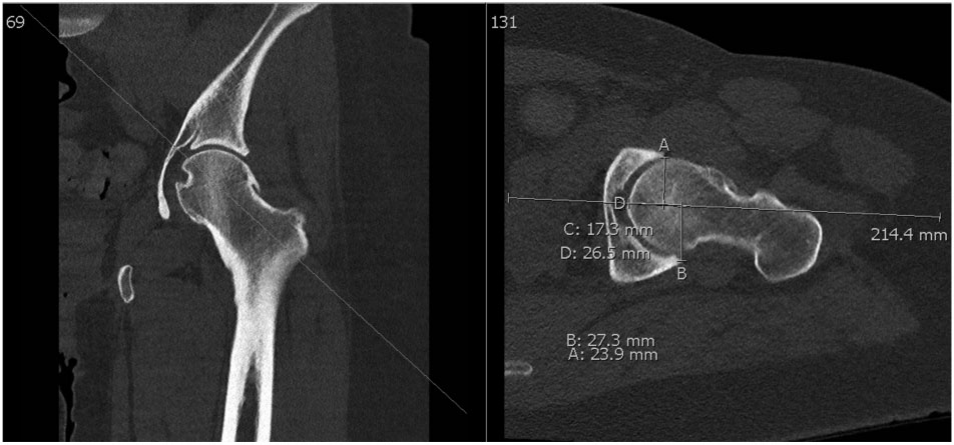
CT coronal and CT swiss axial cross sections imaging demonstrating methodology for calculating the AWI and PWI coverages. A reference line is placed through the center of the femoral head. The distance from the femoral head along the reference line to line A represents the AW coverage. This is repeat to line B for posterior wall coverage. This is represented by lines C and D in the image below (anterior wall coverage and posterior wall coverage, respectively). Line D is on top of line C. To calculate the AWI/PWI the AW and PW is divided by the radius of the femoral head.
